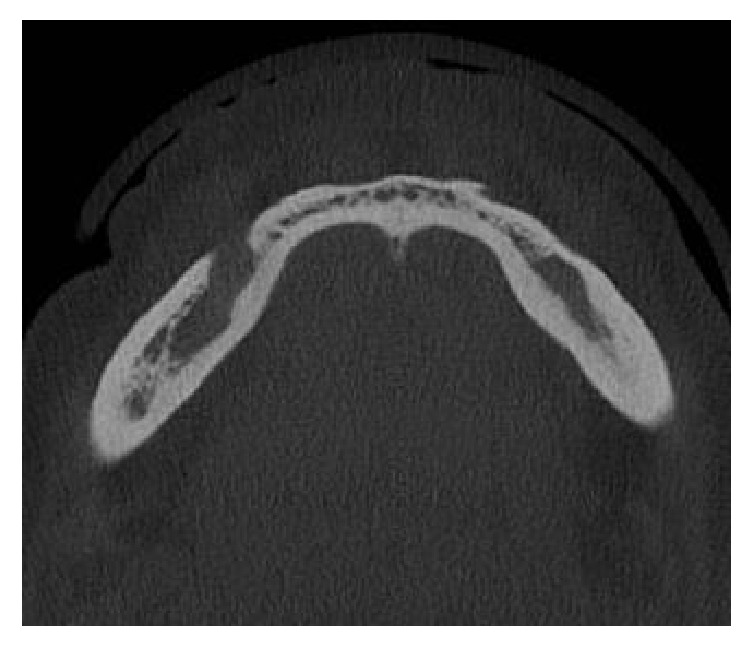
CBCT axial slice demonstrating rupture of the mandibular cortex.
